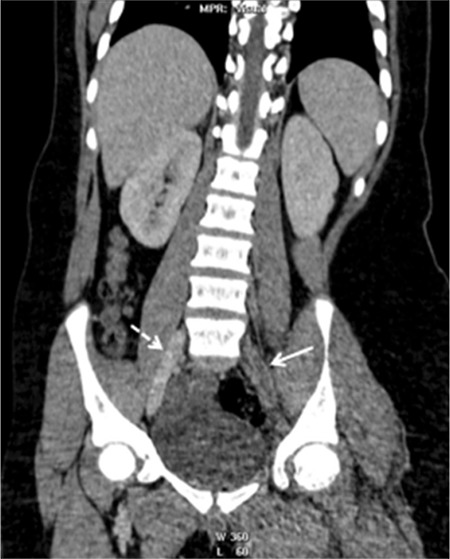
Abdominal computed tomography of the patient demonstrating iliac vein thrombosis (arrows).
